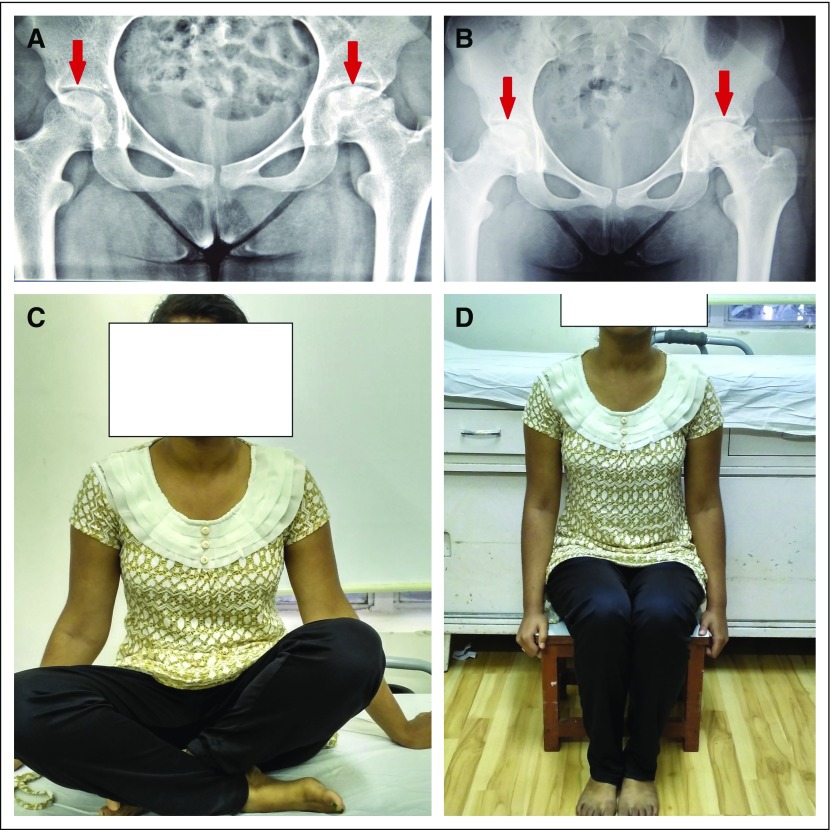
(A) Radiograph of a 19-year-old patient showing Ficat-Arlet stage II avascular necrosis (AVN) right femoral head and stage III AVN left femoral head at the commencement of therapy. (B) Radiograph at year 4 of follow-up demonstrating no radiologic progression of the AVN stage on both the sides. (C and D) Patient is able to squat and sit on a low stool, showing no limitation of daily activities.
